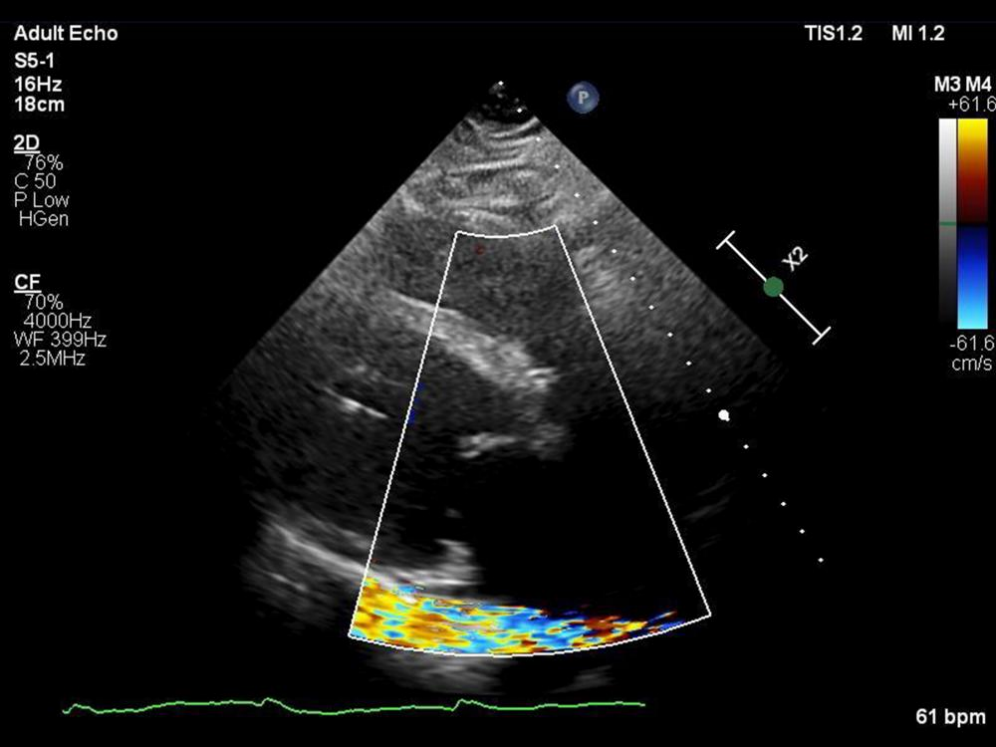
Parasternal long-axis view on transthoracic echocardiography, June 2022, demonstrating resolution of aortic incompetence 2 years and 7 months post-bioprosthetic aortic valve replacement.
